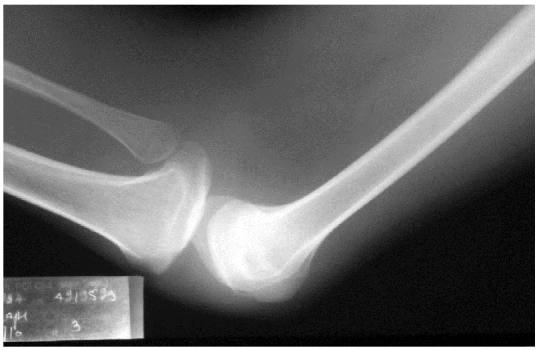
X-Ray of the knee showing absent of patella
